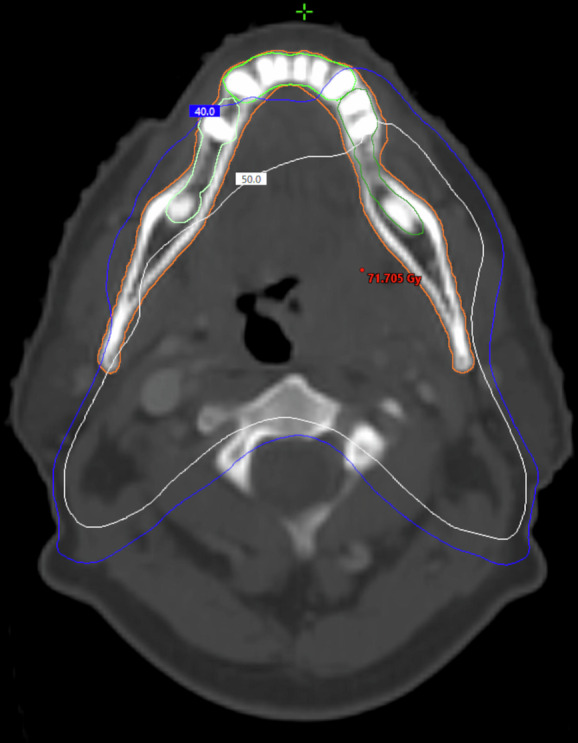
CT scan of patient with oropharyngeal cancer who received bilateral RT with 70 Gy. The figure shows that anterior region is almost completely spared from 40 Gy radiation dose.
